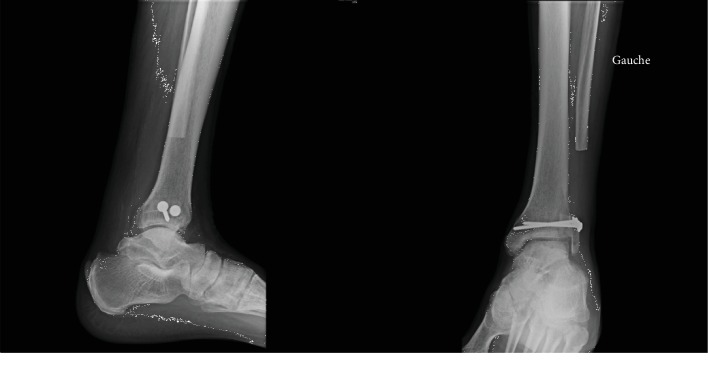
One-and-a-half-month postoperative radiography.
